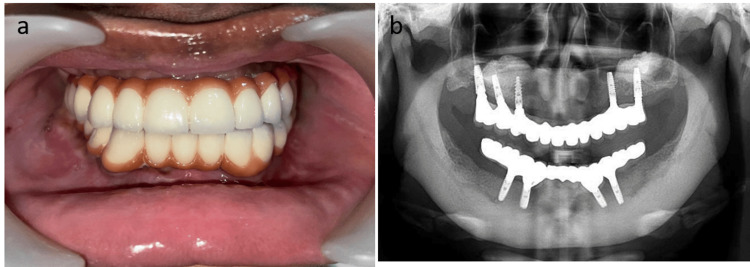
a) Intraoral image showing done implant prosthesis. b) Six months postoperative radiograph of the implant placement
